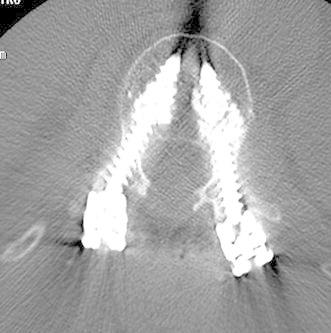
Postoperative CT scan showing the cement extruded around the screws
